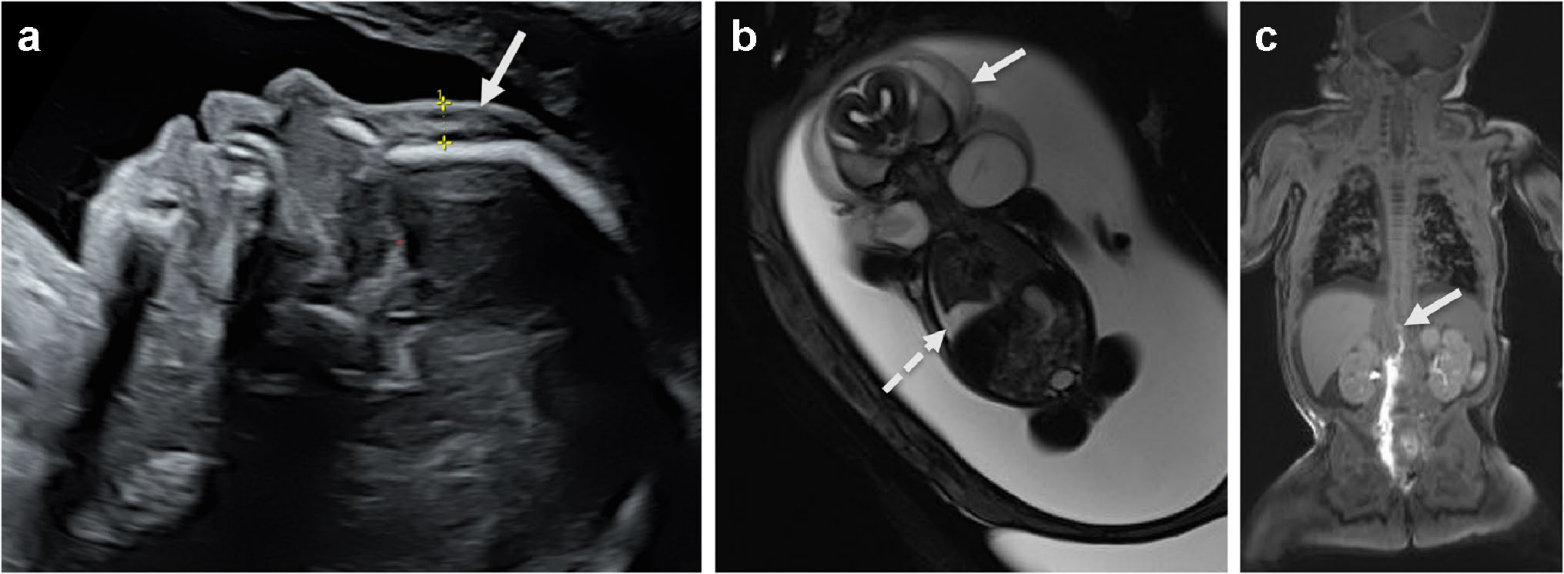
Complex lymphatic anomaly cases. a, b Prenatal sagittal ultrasound (a) and coronal magnetic resonance (b) images of a male fetus at 23 weeks gestational age. a Demonstrates extensive subcutaneous edema (*arrow*) with the calipers measuring 8 mm thickness. b Demonstrates extensive subcutaneous edema (*arrow*) and pleural fluid (*broken*
